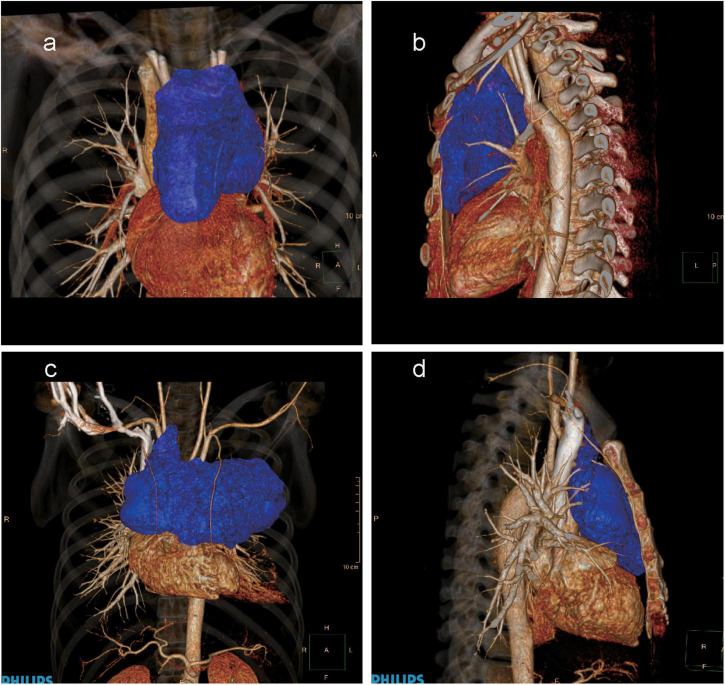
Three-dimensional computed tomography (CT) images of the large anterior mediastinal mass. (a) The anteroposterior CT image of the first patient (tumor represented in blue). (b) The lateral CT image of the first patient (tumor represented in blue). (c) The anteroposterior CT image of the second patient (tumor represented in blue). (d) The lateral CT image of the second patient (tumor represented in blue).
